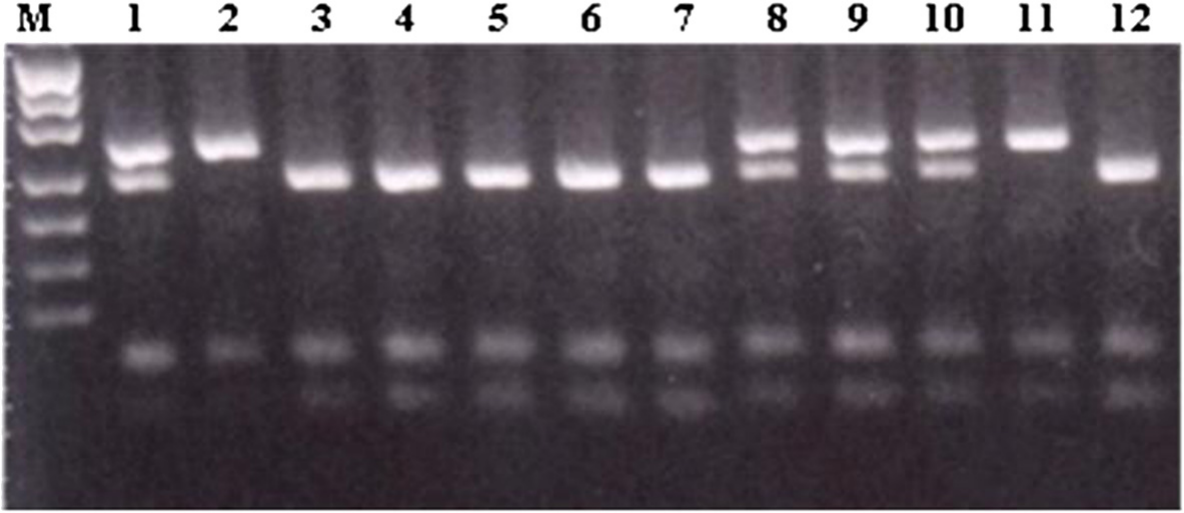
Identification of rs2476601 polymorphism of PTPN22 gene. Lanes: M – DNA length marker (Puc Mix Marker 8, MBI Fermentas), 1, 8, 9, 10 – CT heterozygotes, 2, 11 – TT homozygotes, 3, 4, 5, 6, 7, 12 – CC homozygotes.
